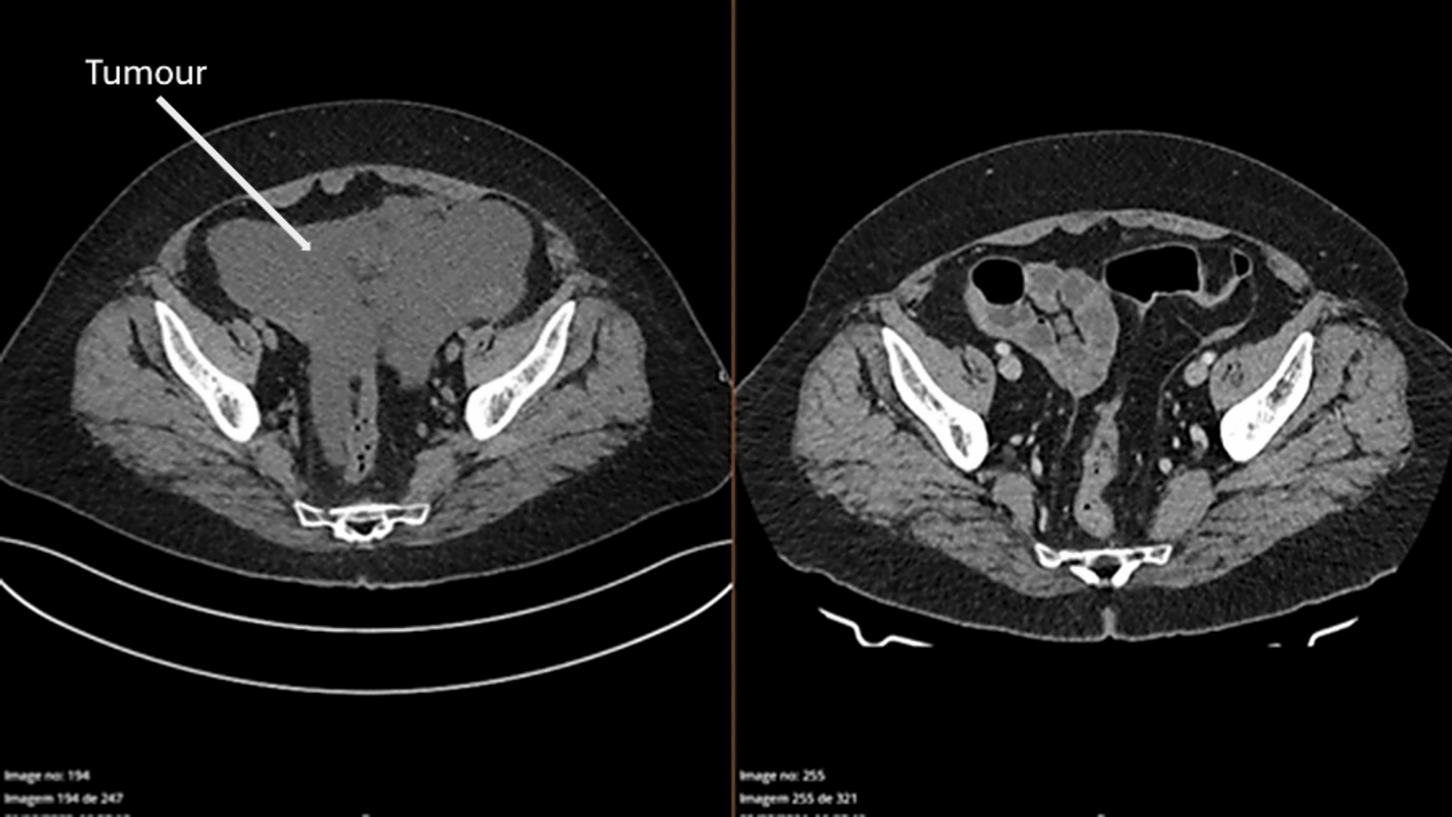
CT scan of the disease before and after chemotherapy Left: before chemotherapy; right: after chemotherapy
